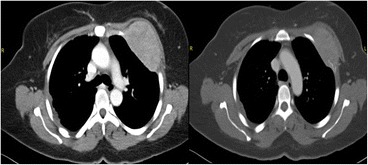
Transaxial images of the contrast-enhanced CT scan of the chest. *Left*: mediastinal window. *Right*: bone window shows an aggressive, destructive bone lesion with a large soft-tissue component arising from the 3rd anterolateral rib, which invaded the adjacent pleural surfaces and the ipsilateral major pectoral muscle
